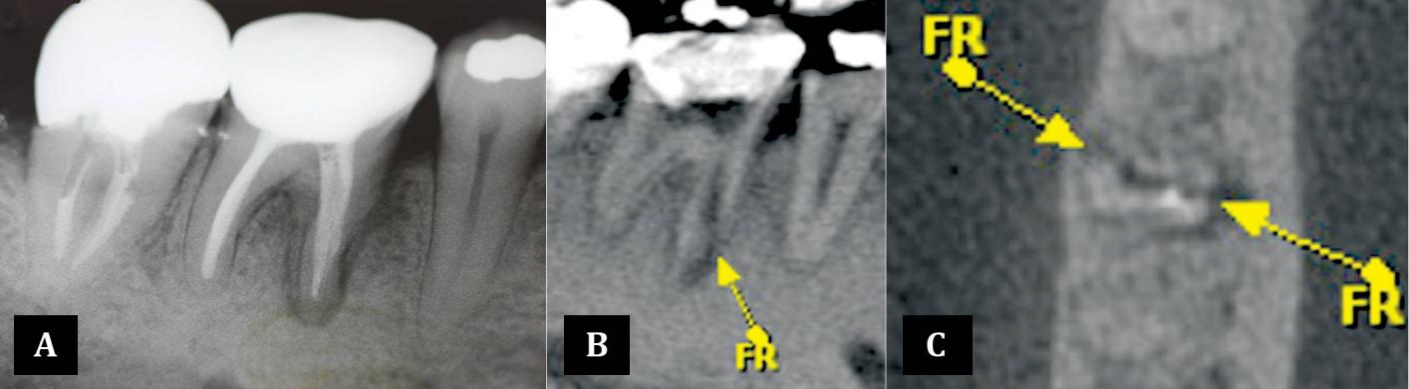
A) Periapical radiograph of tooth 46, showing radiolucent area on mesial side of the root (white arrow); B,C) CBCT images in the sagital and axial planes of the tooth, showing clearly the root fracture (FR) with separation of the fragment
